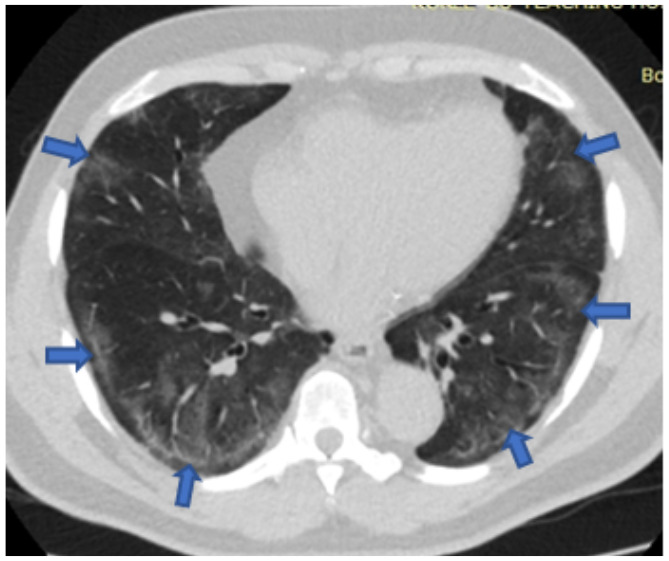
An axial non-contrast enhanced chest CT scan of a 62-year-old man with fever and breathlessness for 5 days. Lung window image shows diffuse bilateral ground glass opacities (Arrows) in a predominantly peripheral distribution. Typical features. RT-PCR confirmed COVID-19.
